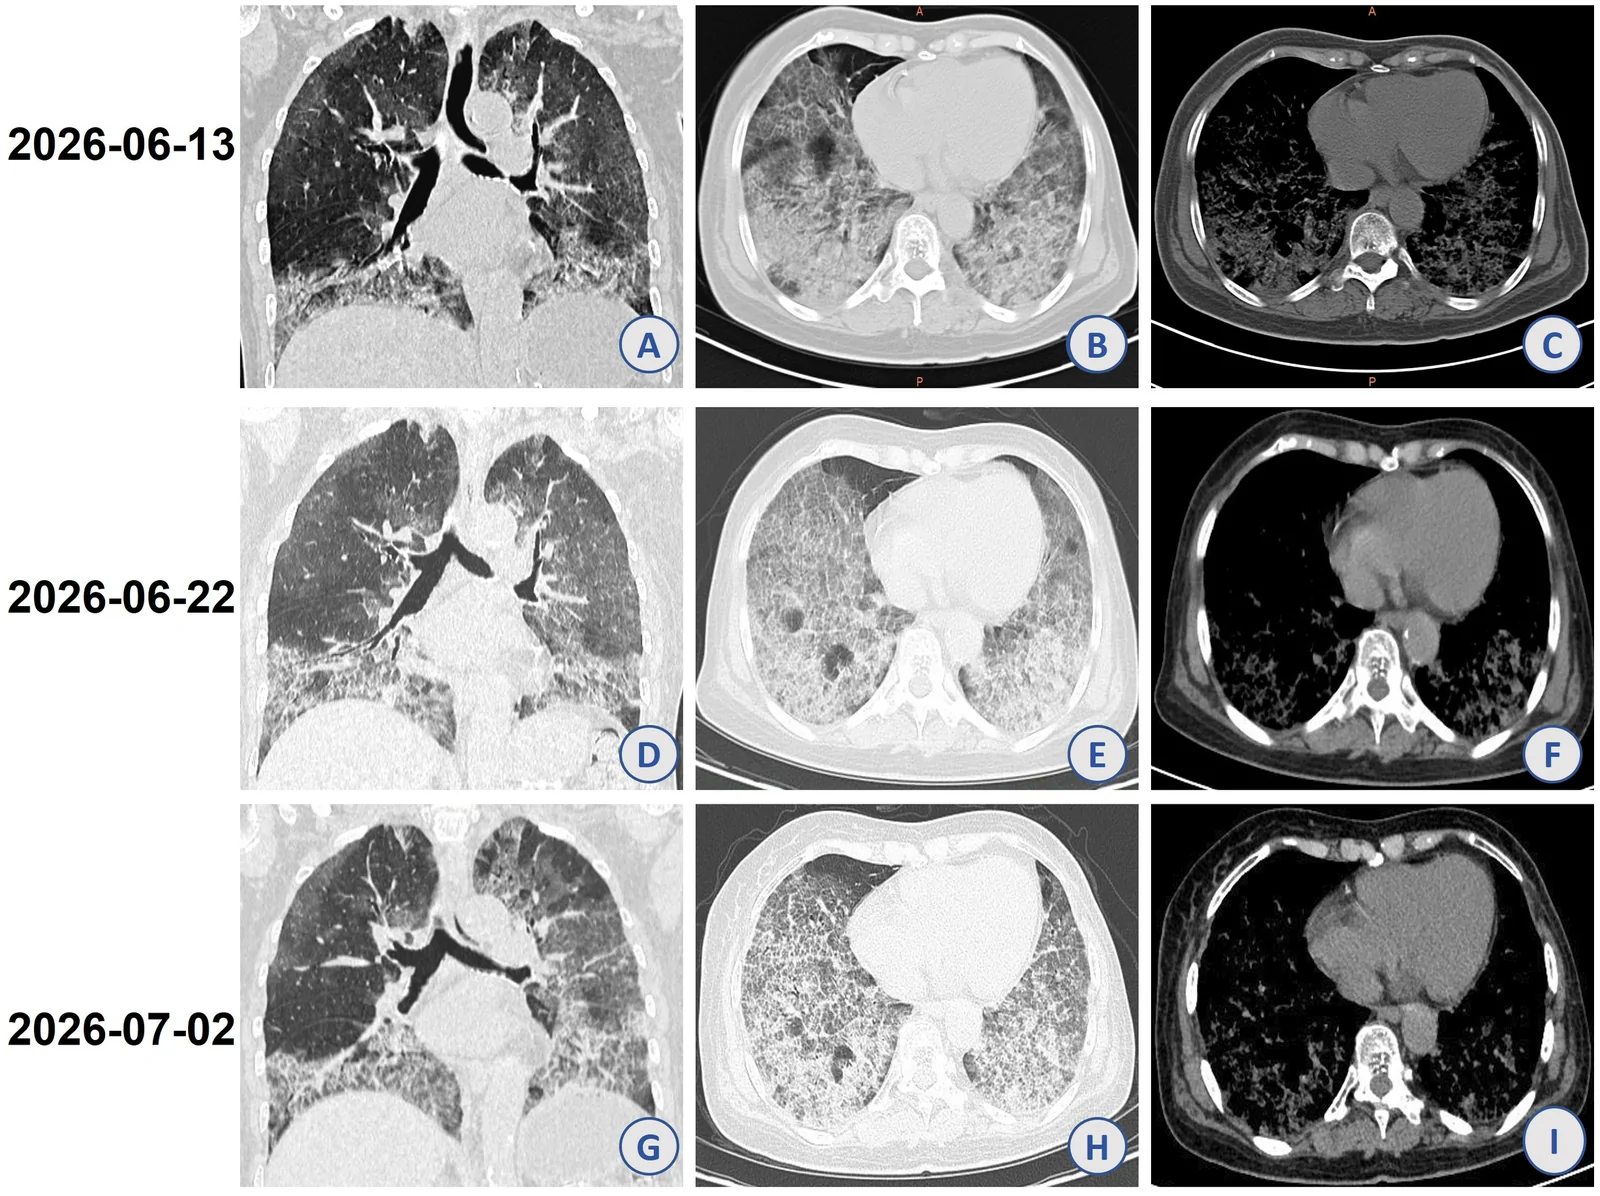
Evolution of chest CT imaging in the patient. Row 1 (A–C): On admission (June 13, 2026), coronal view (A), axial lung window (B), and axial mediastinal window (C) showed diffuse bilateral high-density opacities with interlobular septal thickening. Row 2 (D–F): Follow-up after one week of methylprednisolone 40 mg/d (June 22, 2026), coronal view (D), axial lung window (E), and axial mediastinal window (F) demonstrated no significant resolution of the bilateral lesions. Row 3 (G–I): Re-examination after the addition of mycophenolate mofetil and nintedanib (July 2, 2026), coronal view (G), axial lung window (H), and axial mediastinal window (I) showed still no change in the pulmonary lesions.
